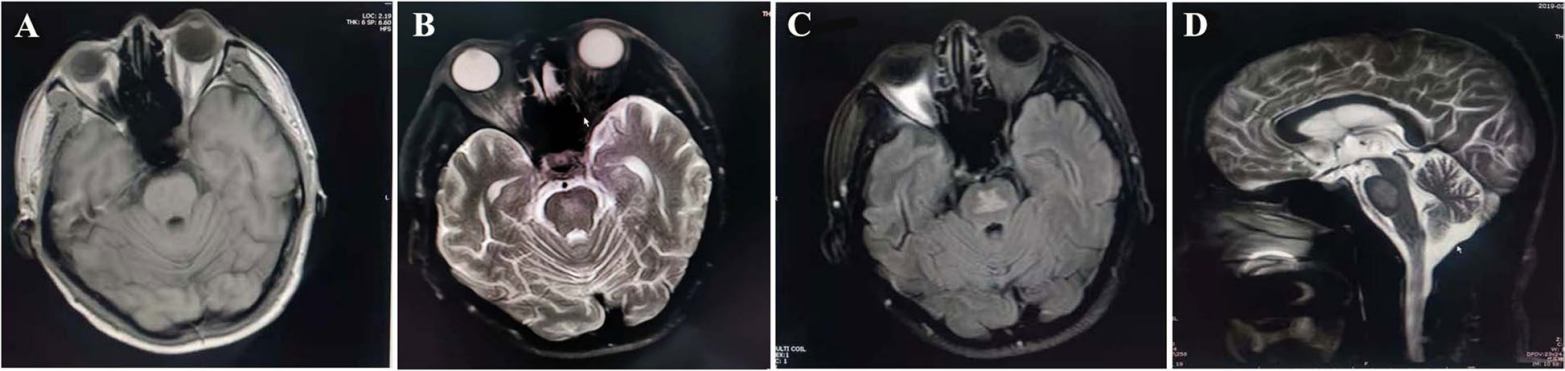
MR images of the brain. A T1-weighted imaging showing a mildly hypointense lesion in the central pons; (B, D) T2 and (C) FLAIR-weight imaging demonstrating a hyperintense lesion in the same location
